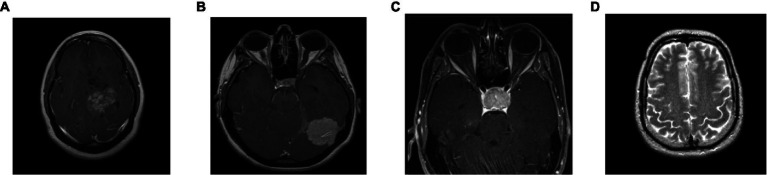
Sample brain MRI images: (A) glioma; (B) meningioma; (C) pituitary; (D) healthy.
